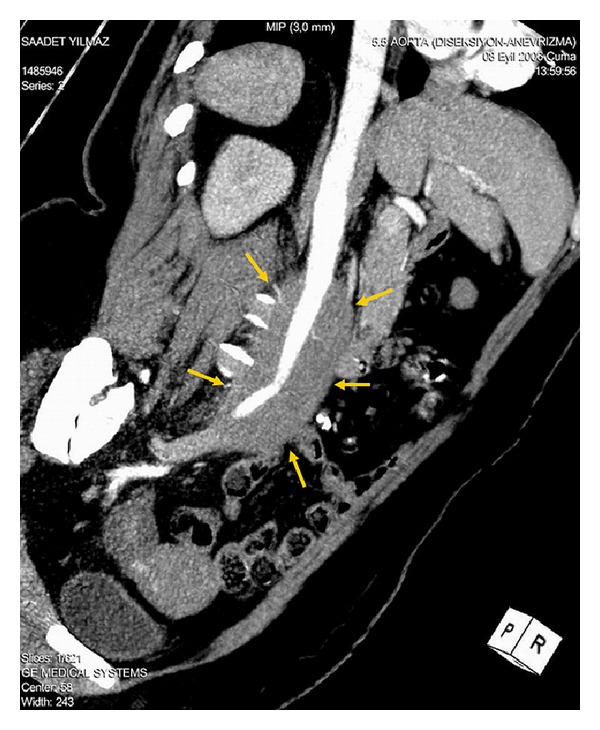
Preoperative contrast-enhanced axial CT images demonstrate that the aorta is surrounded by a soft-tissue mass (yellow arrows).
